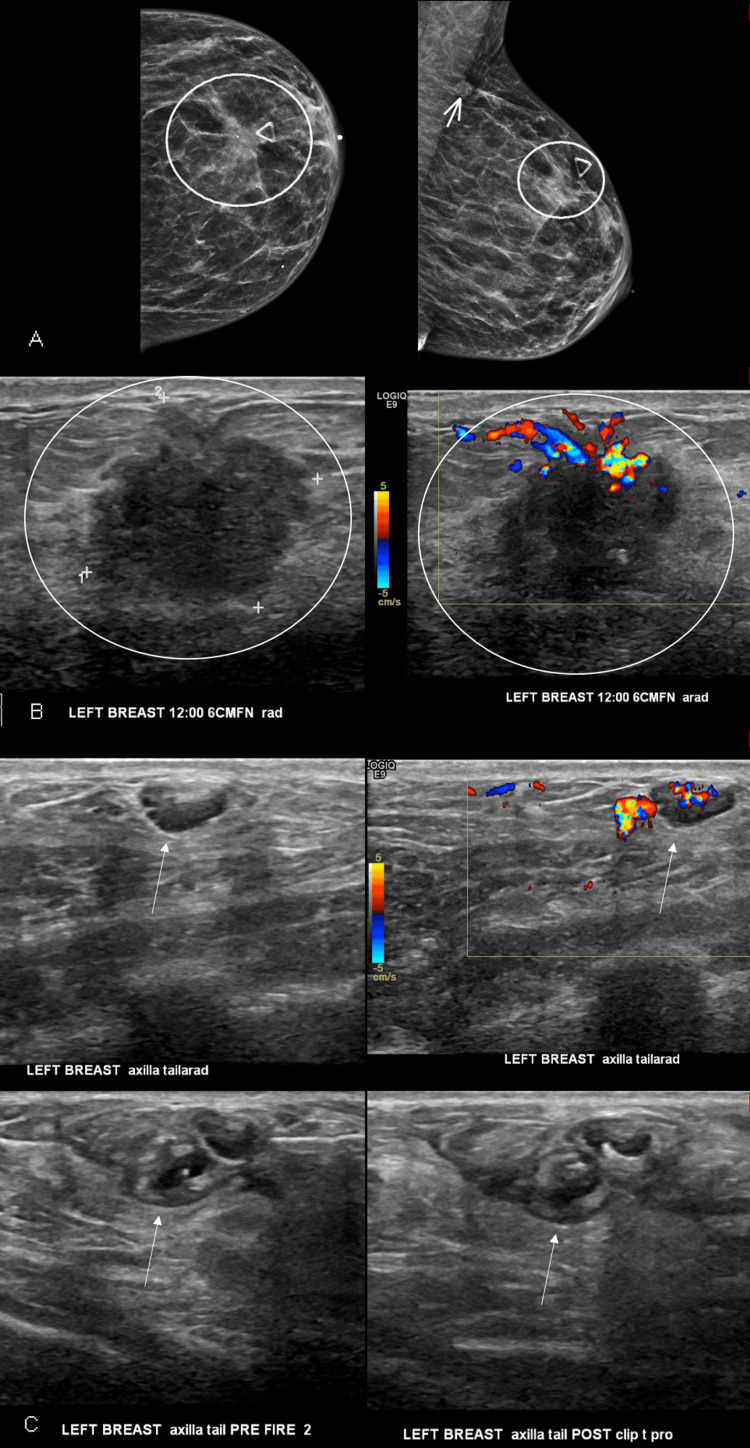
Initial diagnostic imaging and biopsy (A) Diagnostic mammogram left craniocaudal (CC) and left mediolateral oblique (MLO) images, left and right images, respectively, demonstrates an upper breast spiculated mass (circle) and axillary tail asymmetry (arrow). (B) Diagnostic ultrasound demonstrated correlates for the mammographic findings, including a spiculated mass at “left breast 12:00 6 cm FN” (top row, circles) and lymph node within the “left breast axillary tail” (bottom row, arrows). (C) Subsequent ultrasound guided biopsy demonstrates, in sequential order from left to right, bleeding of the lymph node noted immediately after the first pass and prior to the second pass, followed by imaging of the lymph node at clip placement (arrows).
